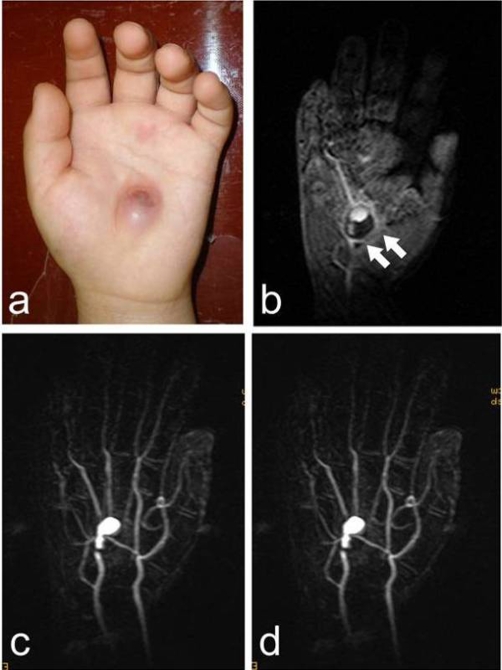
A pulsated mass in the palm can be seen (a). The T1-weighted MRI image shows a low-signal intensity area suggesting a thrombus within the lesion (arrows) (b). A stereoscopic MRA image shows an aneurysm in the superficial palmar arch (c, d).
